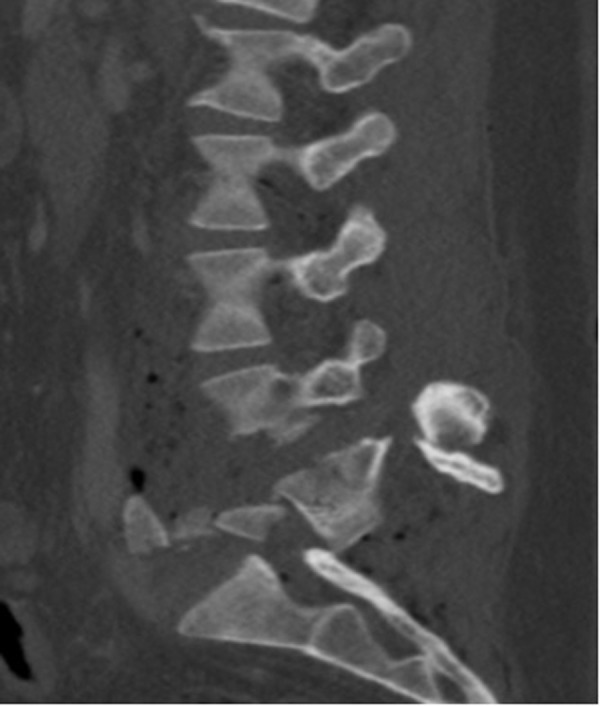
Preoperative parasagittal CT This is a preoperative parasagittal CT demonstrating a fracture dislocation with posterior retrolisthesis of the L5 vertebrae over S1.
